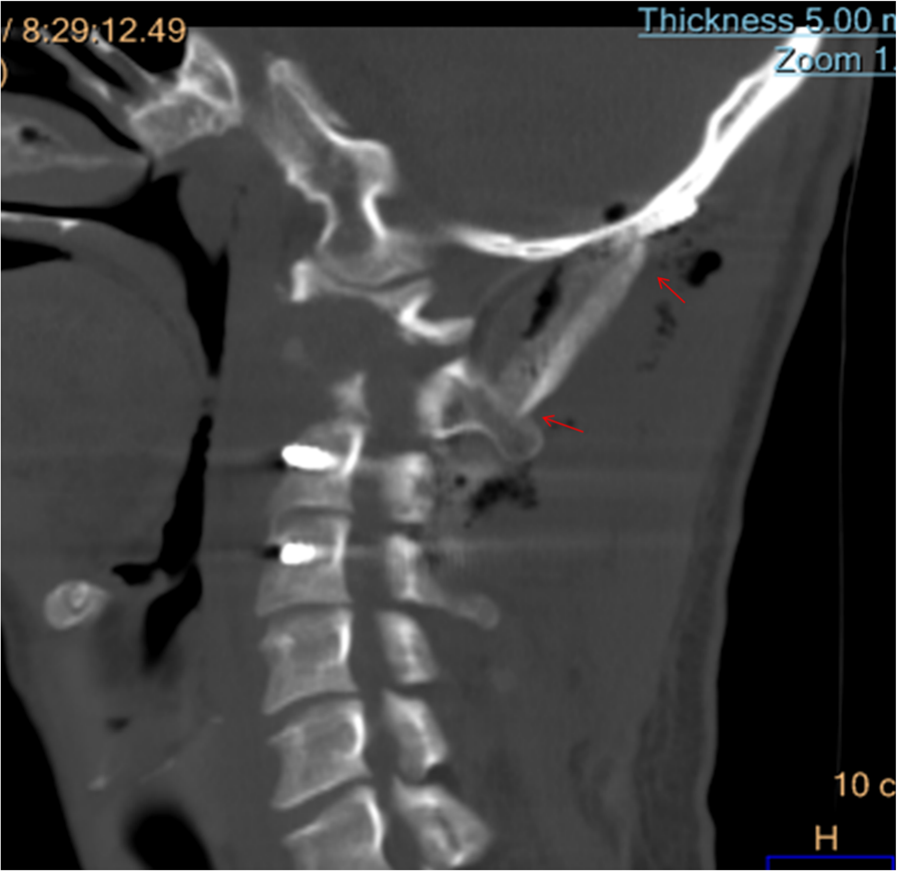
CT examination 9 months after operation showed occipitocervical fusion by posterior bone graft
